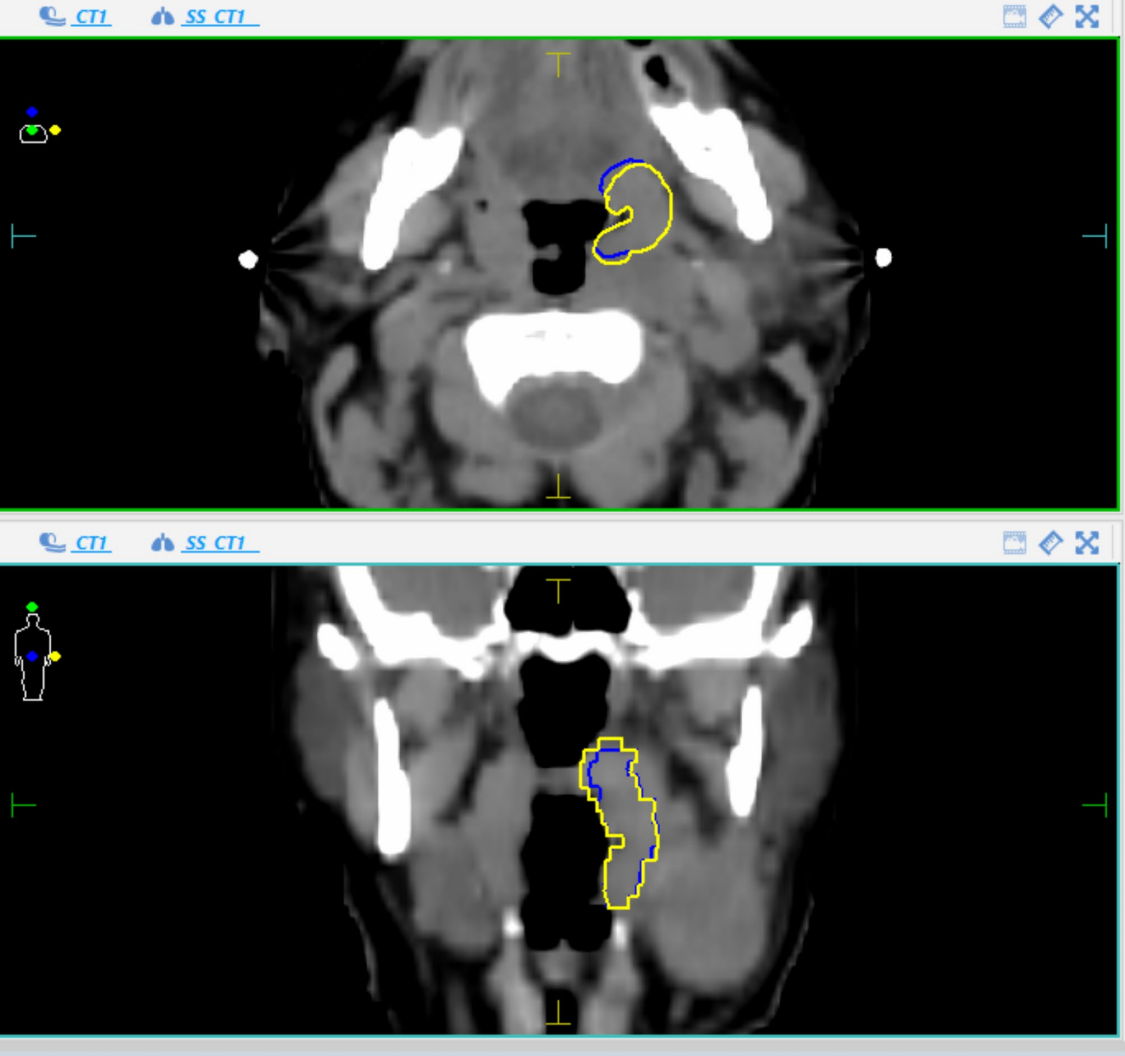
An example of “no relevant change”. Blue = CT contour, Yellow = MRI contour
